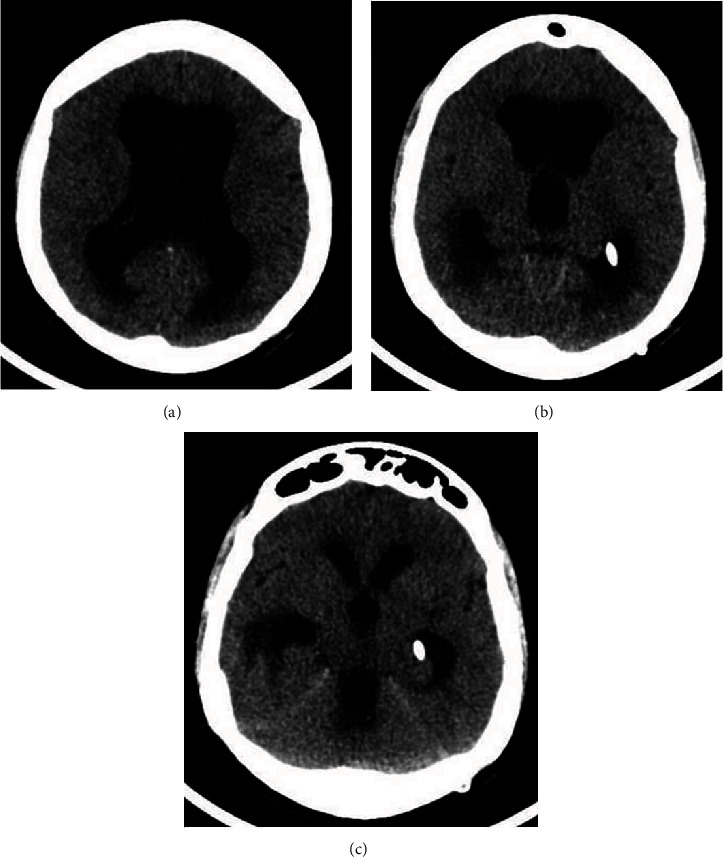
Preoperative cranial CT. (a–c) Axial slices demonstrate ventriculomegaly, enlargement of the temporal horns, and transependymal edema suggesting VPS obstruction. The ventricular catheter is positioned inside the temporal horn.
